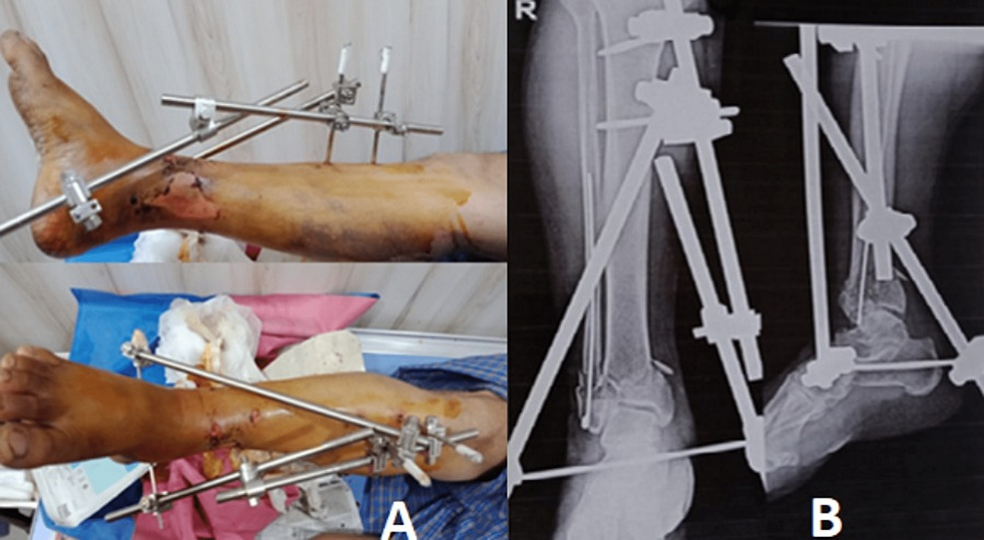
Immediate post-operative clinical image and X-rays (Step 1) A: Clinical image of the delta fixation B: Post-operative X-rays in anteroposterior and lateral views
